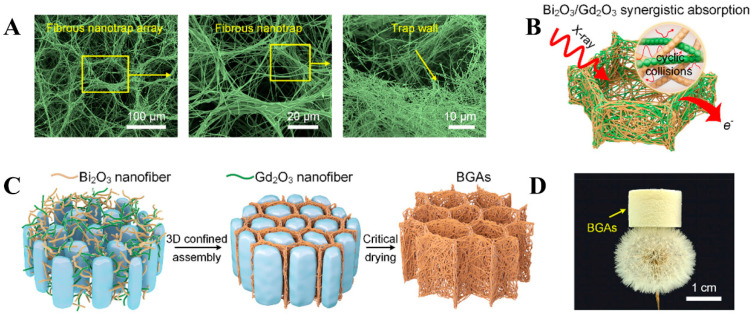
(A) Morphologies of BGAs. (B) X-ray shielding process of BGAs. (C) Fabrication process of the BGAs. (D) BGAs standing on a dandelion. (A–D) Reprinted with permission from Ref. [118]. Copyright 2023, American Chemical Society.
